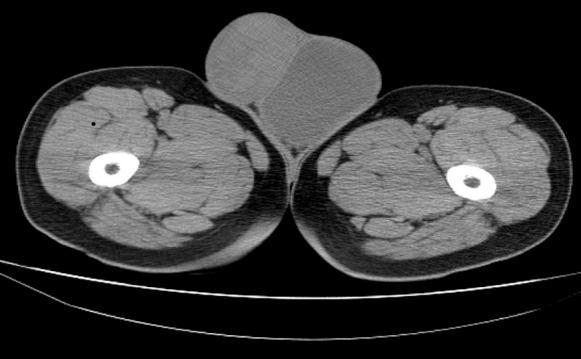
CT image showing bilateral neoplastic testicular enlargement
